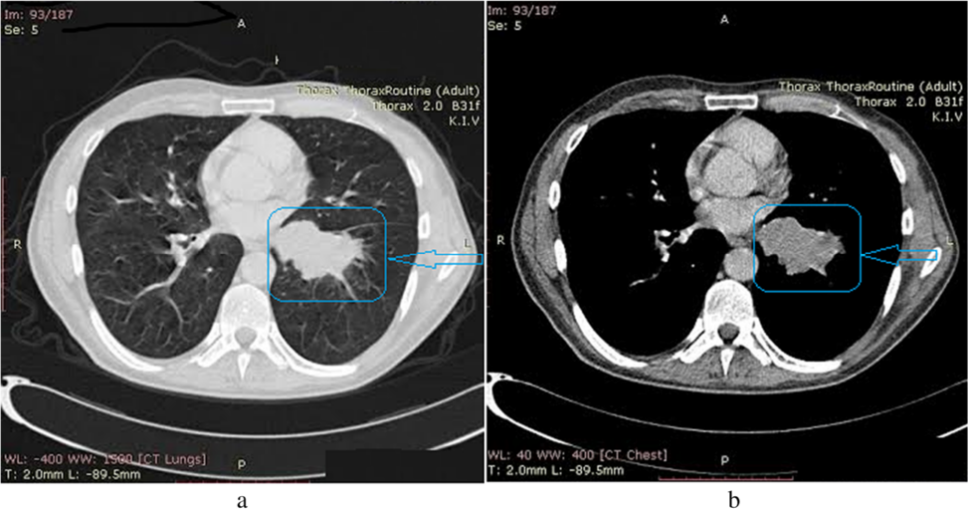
(a, b) Computed tomography revealed an expansive process in the left lung, left hilar and mediastinal lymphadenopathy.
